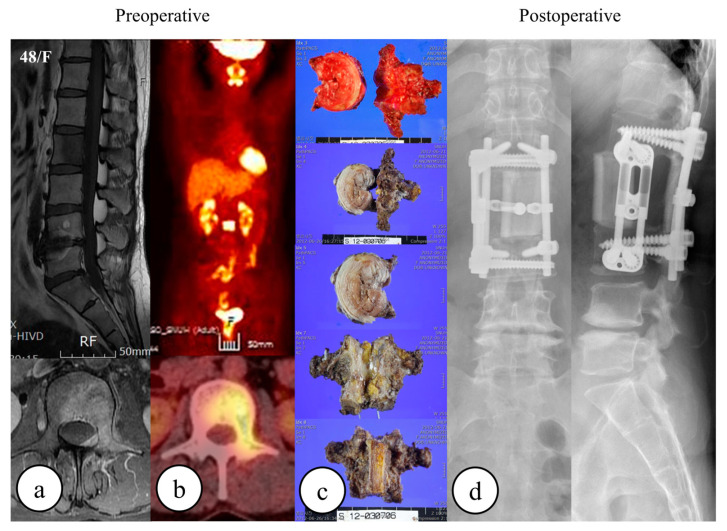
A 48-year-old female underwent total en bloc spondylectomy for a solitary metastasis of breast cancer at L2. The following images are provided: (a) preoperative MRI, (b) preoperative whole-body FDG-PET CT, (c) gross pathology, and (d) postoperative radiography.
